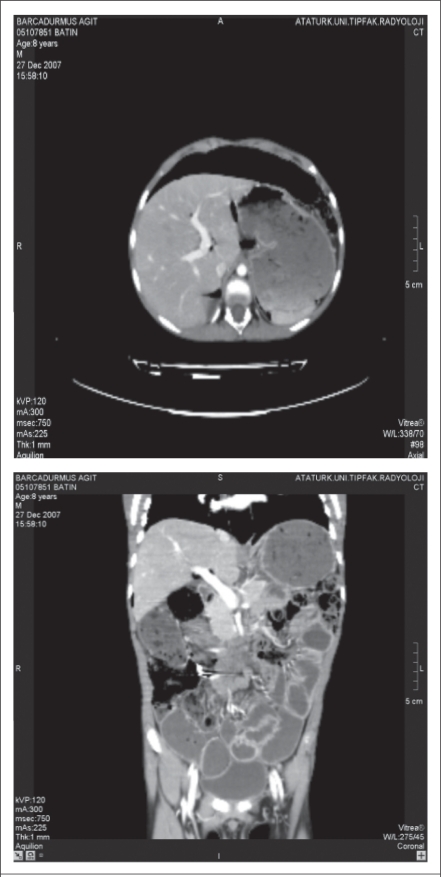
Abdominal CT after treatment
